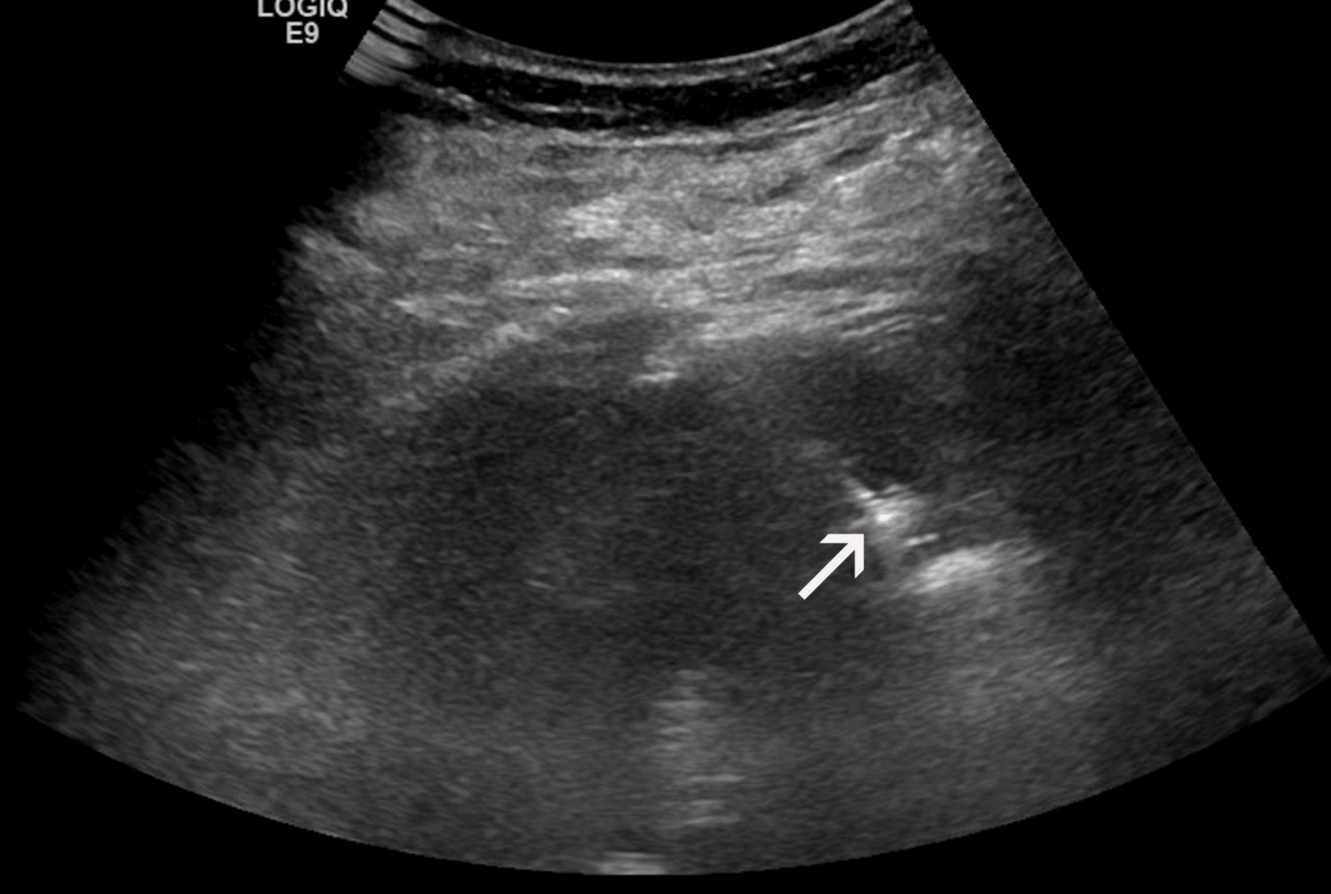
Coronal-oblique ultrasonographic image during microwave ablation (MWA). Image shows the tip of the MW antenna (arrow) at the deepest part of the tumor.
